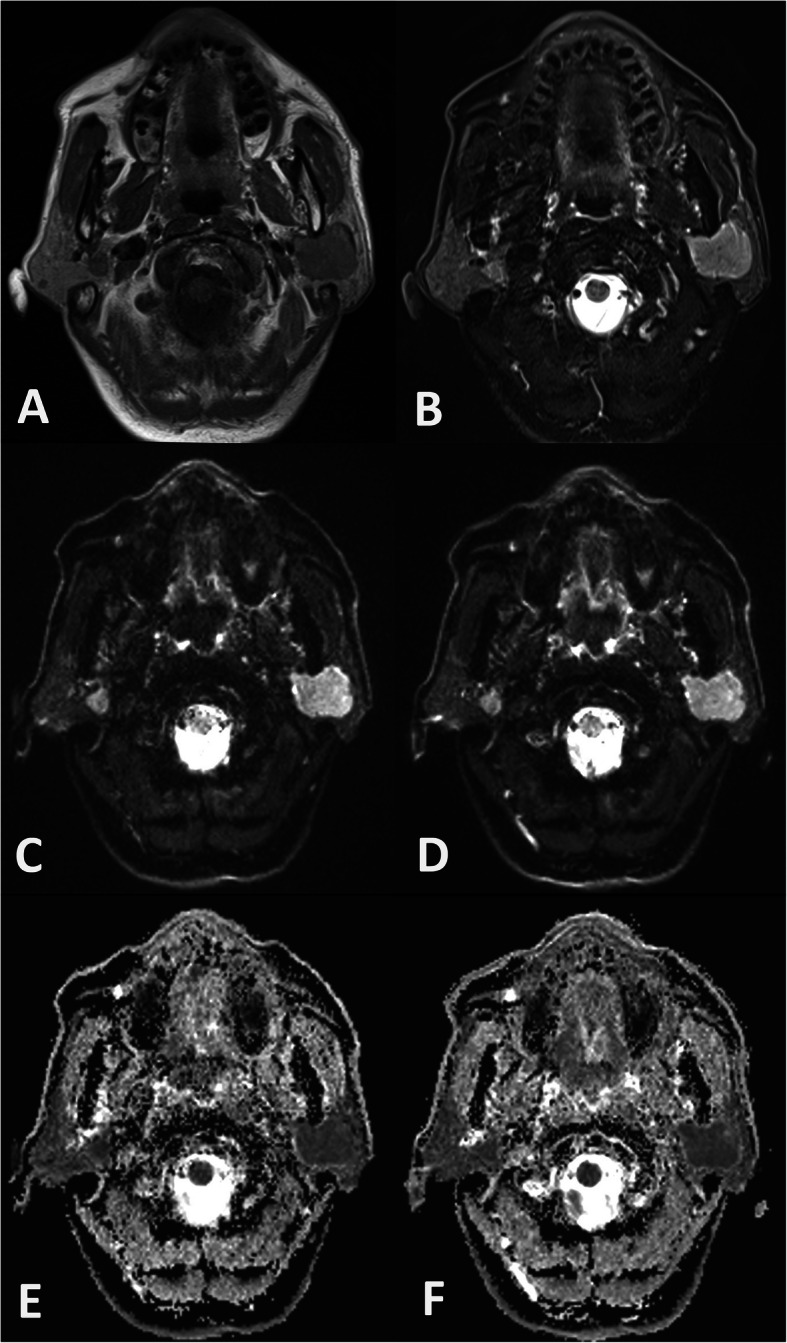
Example of SMS-RESOLVE DWI, RESOLVE DWI, and corresponding ADC maps of a 57-year-old patient with bilateral warthin tumors in parotid glands. The tumor showed slight hyperintensity on T1-weighted image (a), hyperintensity on T2-weighted image (b), and hyperintensity on axial DWI (c, SMS-RESOLVE DWI; d, RESOLVE DWI). Image quality scores were similar for SMS-RESOLVE DWI and RESOLVE DWI. The ADC value of the tumor derived from SMS-RESOLVE DWI (e) and RESOLVE DWI (f) were 0.61 × 10^− 3^ mm^2^/s and 0.62× 10^− 3^ mm^2^/s, respectively
